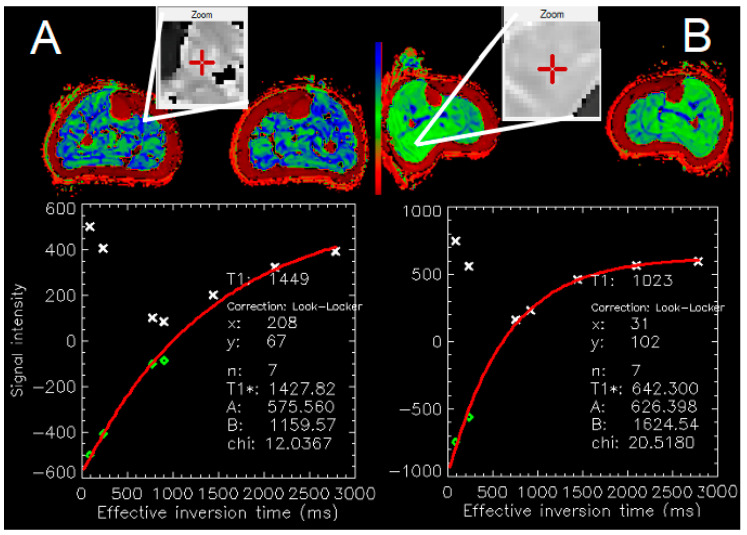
3T MRI MOLLI T1 map (mid-calf level). Panel (A): PAD patient. Zoom area from the soleus muscle. Panel (B): Matched control. Zoom area: soleus/gastrocnemius muscle. Note the significantly higher T1 values in the PAD patient (1449 ms) vs. control (1023 ms). MRMap [20], PRISM color-table.
